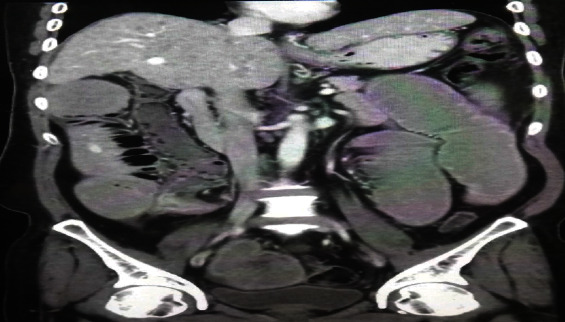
Case 4. Coronal postcontrast CT scan study showed a collapsed cecum with dilated ileum and enhanced lesion at the ileocecal valve.
